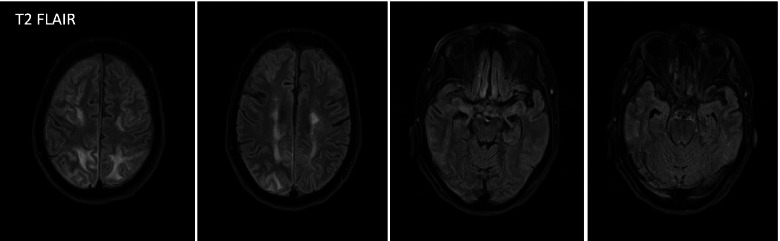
Axial T2-weighted, FLAIR images, showing almost complete resolution of the extensive patchy and confluent hyperintensities in the occipital, parietal lobes, and areas of frontal lobe 3 days later
